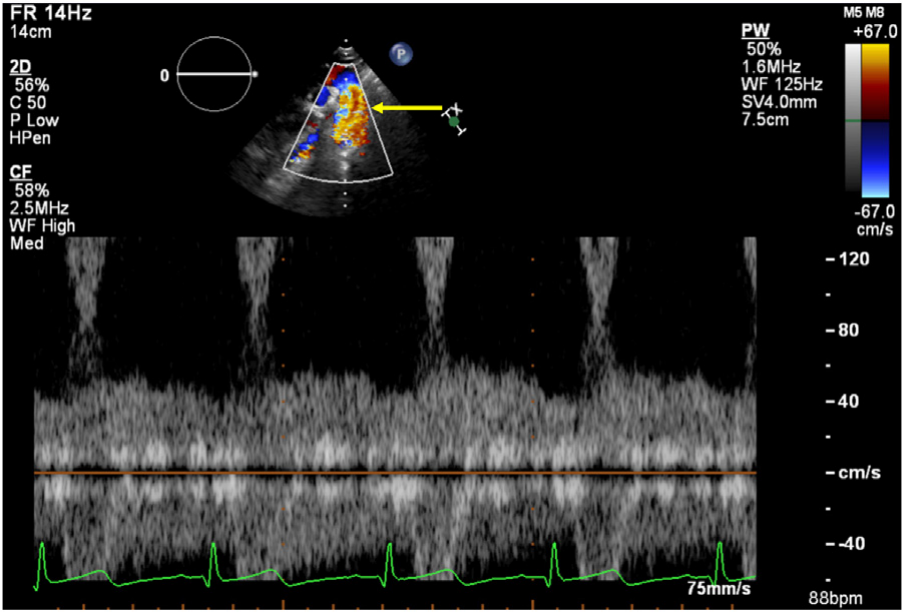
Two-dimensional TTE, suprasternal, sagittal view with color flow–guided continuous-wave Doppler, cursor within the descending aorta *(arrow)*, demonstrates cranially directed flow that may be misinterpreted as aortic regurgitation. However, given the continuous spectral Doppler flow pattern, this likely represents flow from a separate vessel in close proximity to the aorta rather than flow within the aorta itself.
